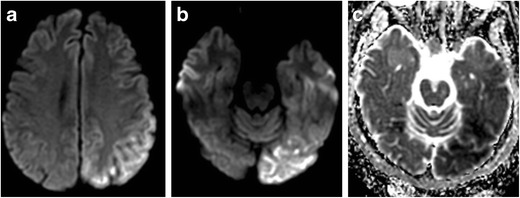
Status epilepticus. Forty-eight-year-old woman with seizures and altered mental status. DWI (a, b) demonstrates a predominantly increased signal with left parietal, occipital, and posterior temporal cortex. ADC (c) demonstrates a corresponding low signal consistent with reduced diffusion. Note that the area of signal abnormality spans both middle cerebral artery (MCA) and posterior cerebral artery (PCA) vascular territories
